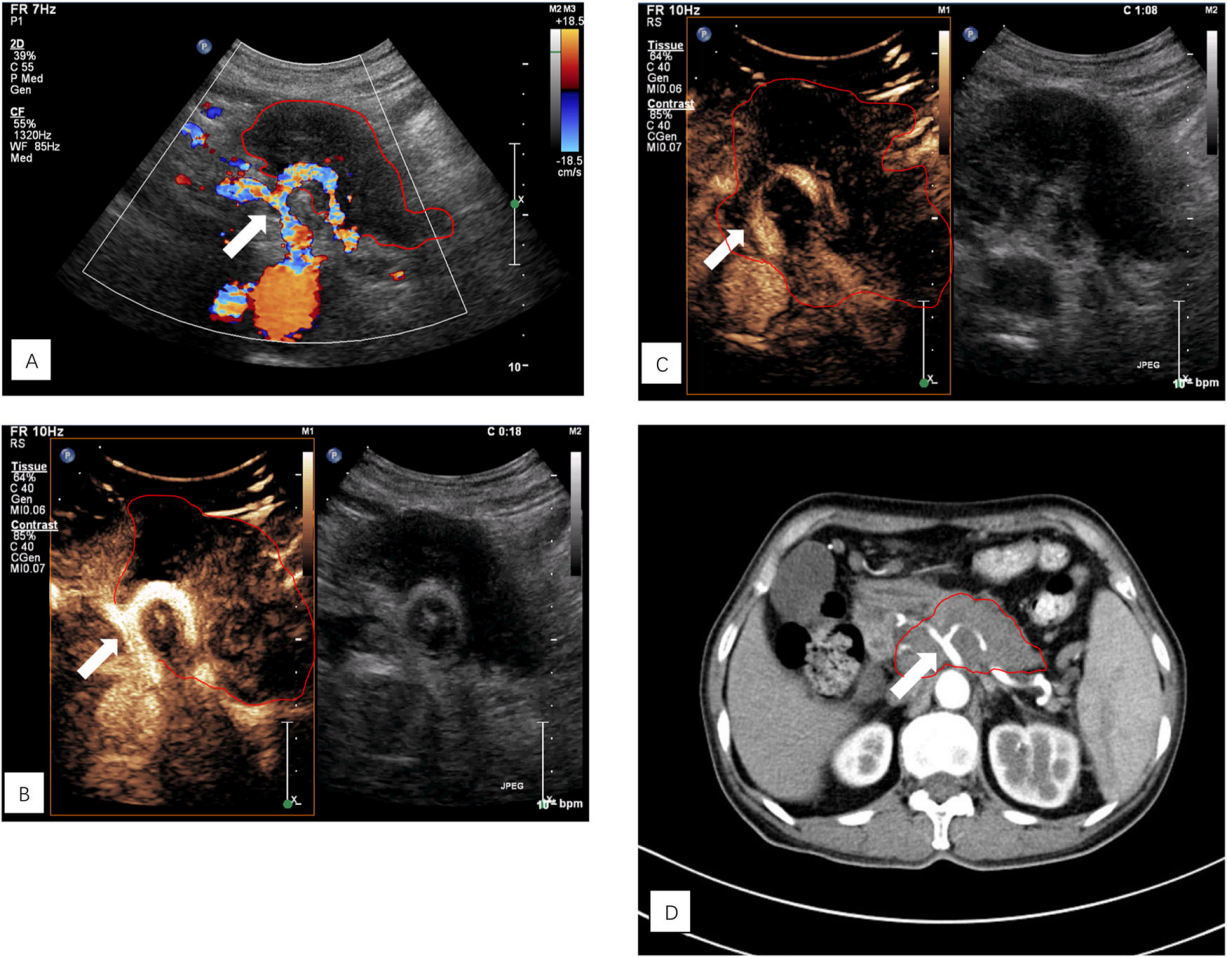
A 58-year-old female patient with PDAC located in the body of the pancreas. A Color Doppler US showed the relationship between the CA (arrow) and the lesion (red border), which was close to but not in contact with the CA. B The arterial phase CEUS scan showed the relationship between the CA (arrow) and lesion (red border), which was in contact with the CA. C The venous phase CEUS scans showed the relationship between the CA (arrow) and the lesion (red border), which completely surrounded the CA. D The arterial phase CECT scan showed that the lesion (red border) was completely wrapped around the CA (arrow)
